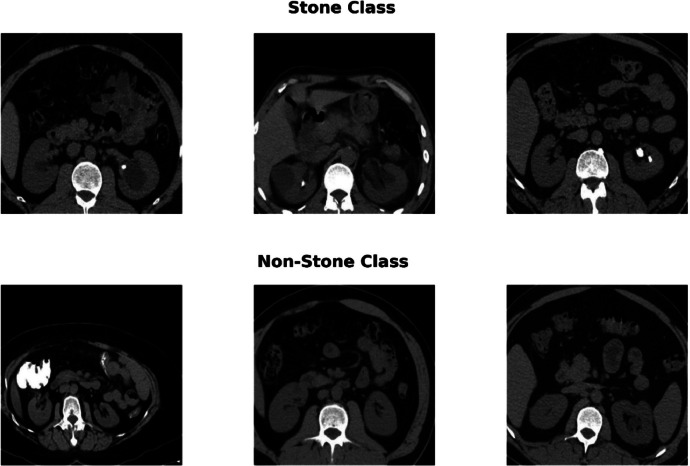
Representative samples of kidney CT images: stone and non-stone classes.
